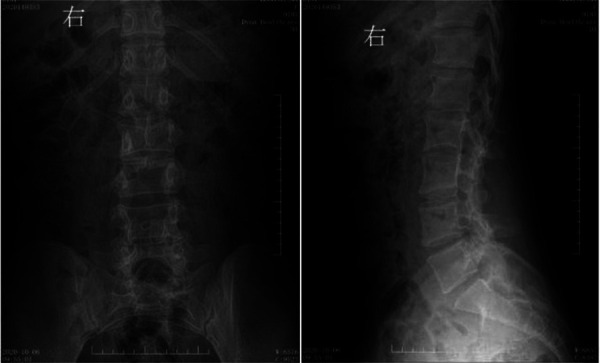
Postoperative digital radiography. The spine was bifida, with no display of some lumbar spinous processes.
